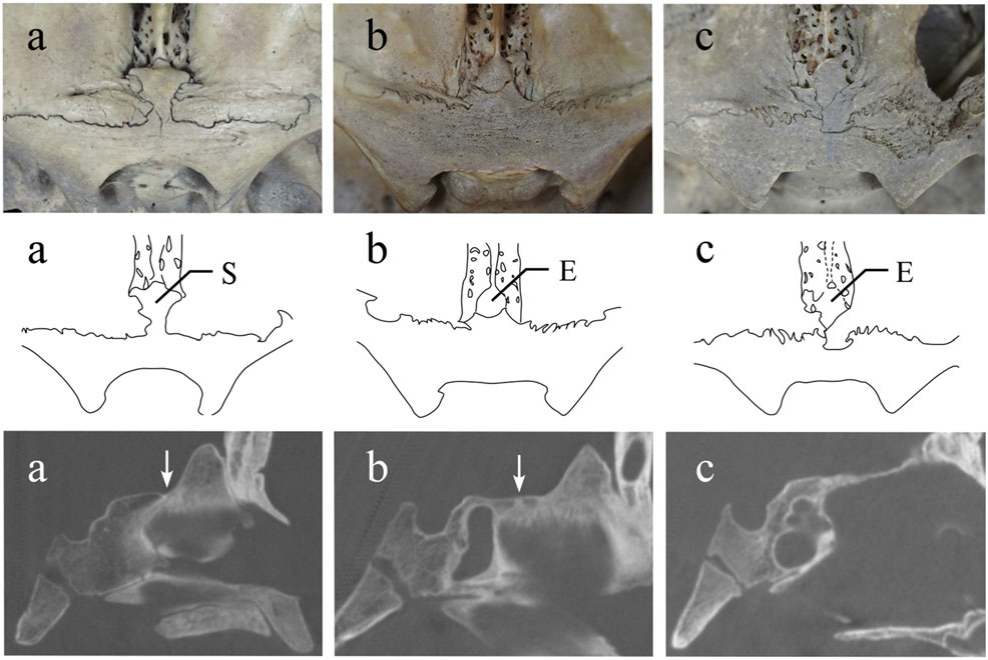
Morphological variations of the anterior cranial fossa in the skulls of adolescents (10 to 17 years). Upper row shows endocranial photographs, middle row shows traced images of photographs, and lower row shows micro‐CT in the median sagittal plane. (a) Protrusion of the sphenoid body projects anteriorly. (b) Anterior border of protrusion of the sphenoid body is slight, and posterior border of the crista galli shows budding. (c) Frontal bone is intervening between the ethmoid and sphenoid bones. Arrows show spheno‐ethmoidal suture on CT images. CT: computed tomography
